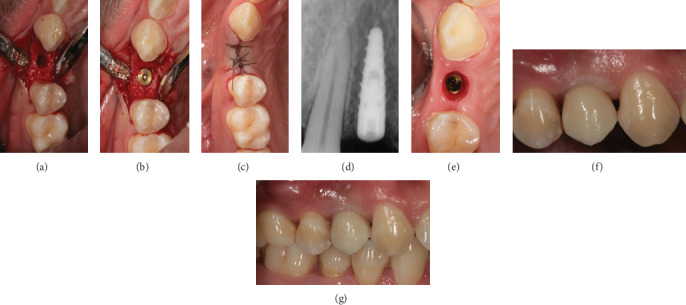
(a) Implant site preparation; (b) implant placement; (c) suture; (d) periapical radiograph after implant placement; (e) soft tissue conditioning; (f) screw-retained zirconia crown; (g) follow-up 6 months after final restoration.
